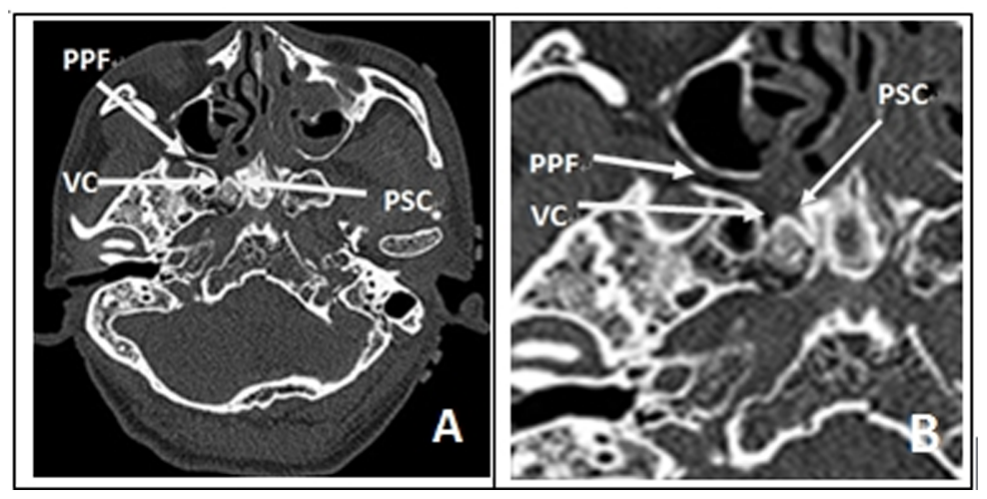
(A&B) Axial CT examinations. It showed relationship of foramen rotundum, vidian canal and palatosphenoidal canal (palatovaginal canal). (PPF: pterygomandibular fossa; PC: vidian canal; PSC: Palatosphenoidal Canal (Palatovaginal Canal); VC: vidian nerve).
